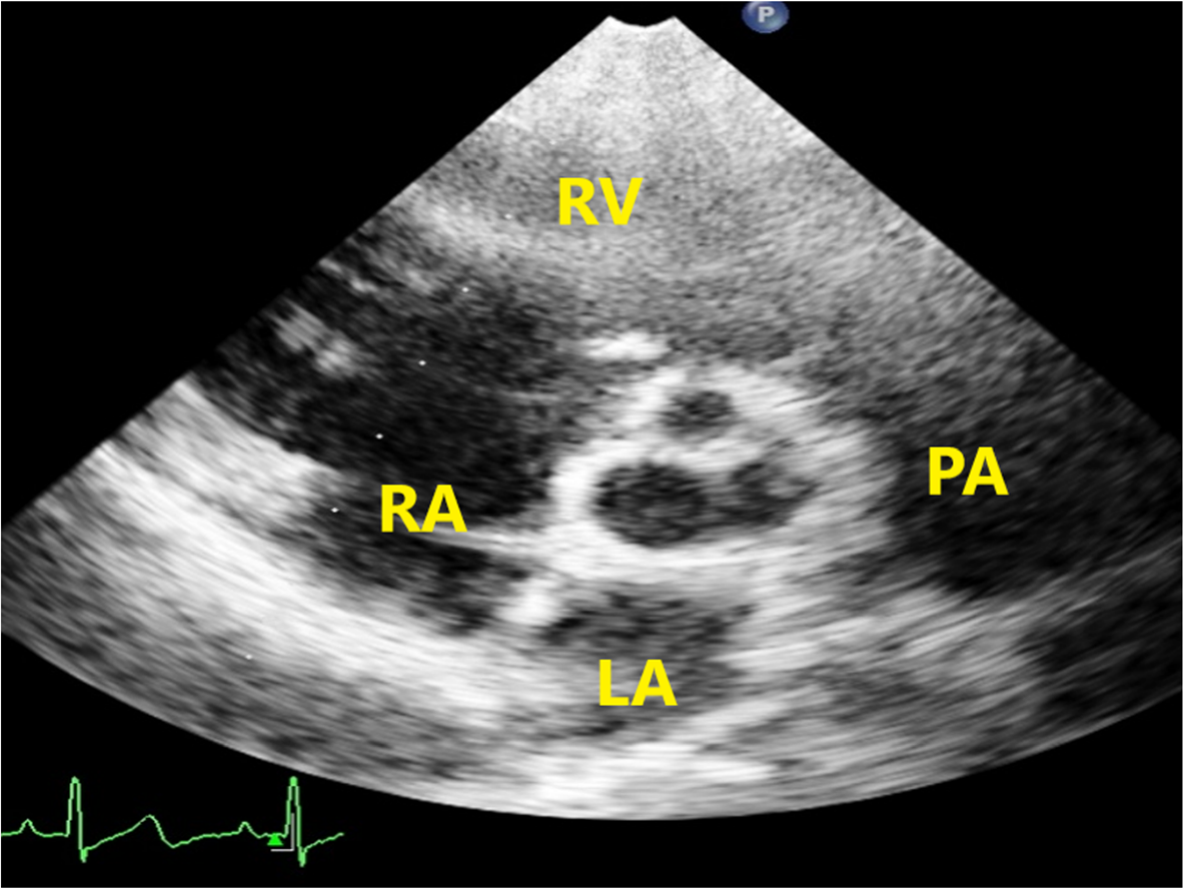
Parasternal short axis view showing the morphology of stenotic aortic valve in patient no. 8
